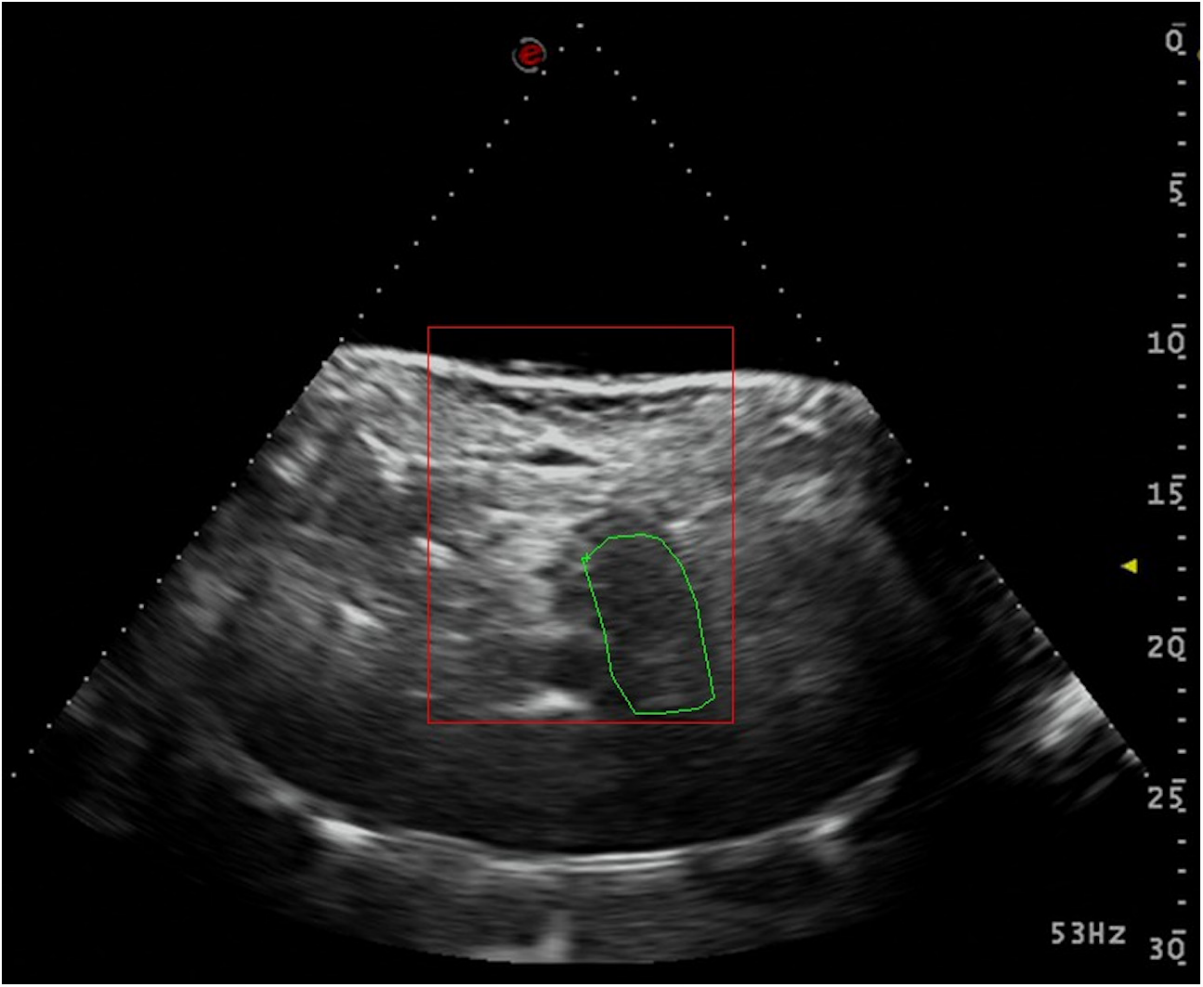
The guiding image during high‐intensity focused ultrasound (HIFU) treatment. The doctor delineated the target treatment area (in the green circle) on the software based on the ultrasound image.
